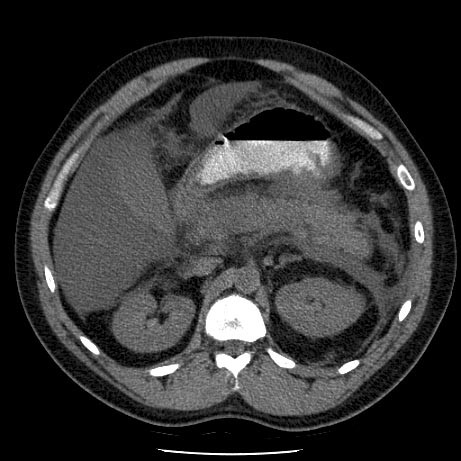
CT scan on admission. Computed tomography on admission shows peripancreatic oedema, thickening of the Gerota's fascia on the left side and at least two poorly defined fluid collections (in other cuts) corresponding to severe acute pancreatitis with Balthazar grade E [21].
